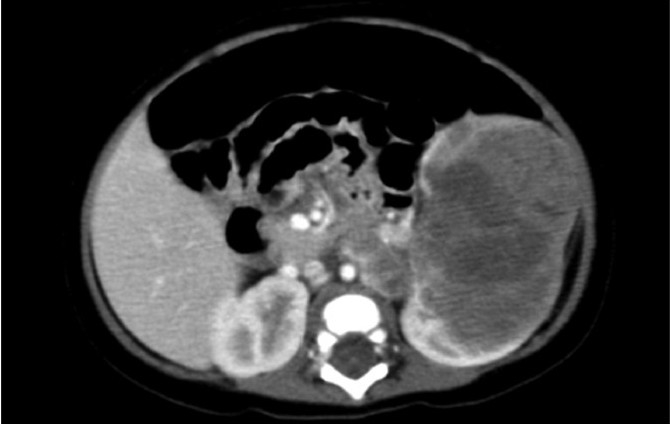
Preoperative abdominal enhanced computed tomography (a large solid mass can be noted in the left kidney; the abdominal lymph nodes were not swollen)
